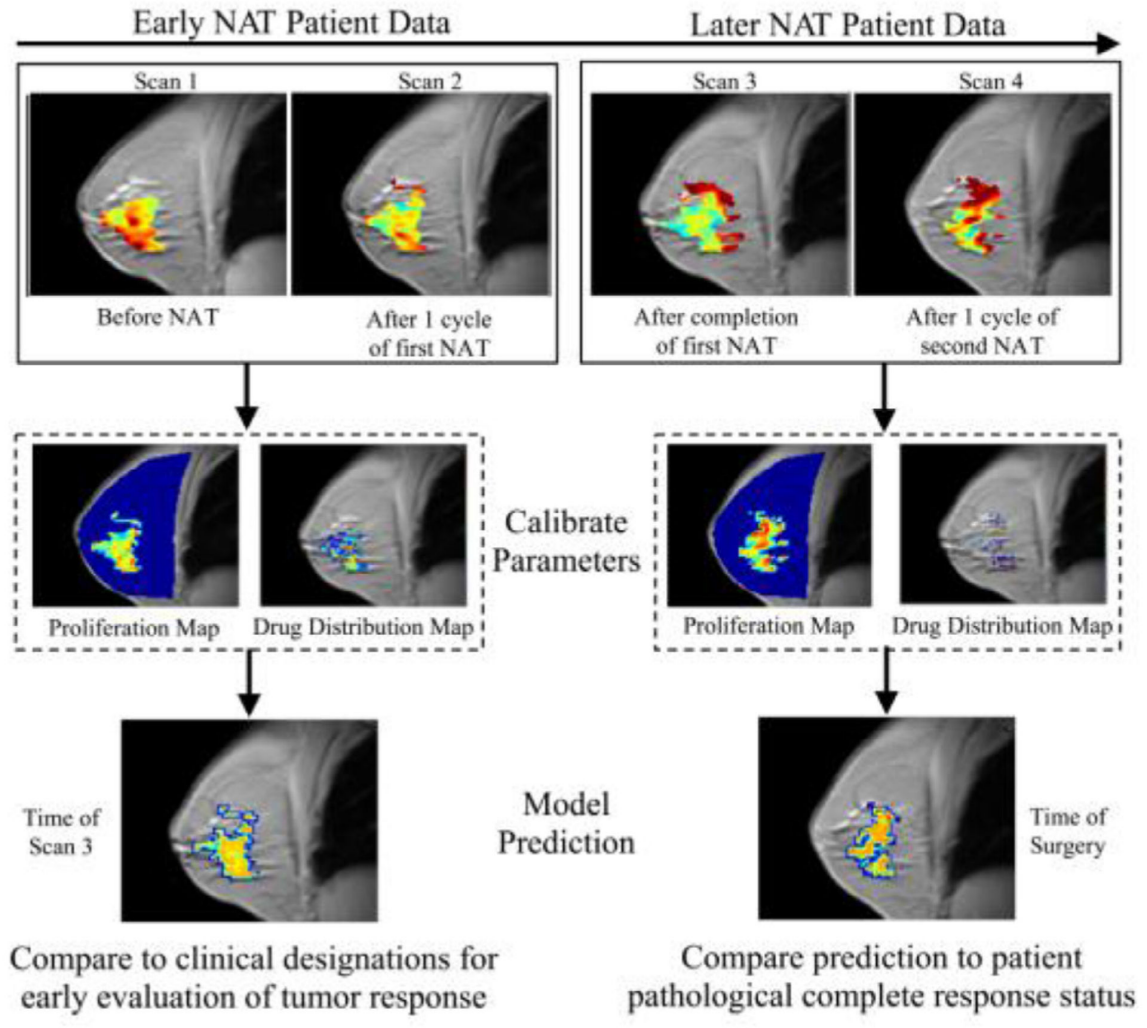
A breast cancer patient was scanned by magnetic resonance imaging at four points during neoadjuvant therapy (NAT). The first two scans (left set of images) are used to calibrate model parameters for predicting response observed at the third time point. The last two scans (right set) are used to update parameters for predicting response observed at the time of surgery.
